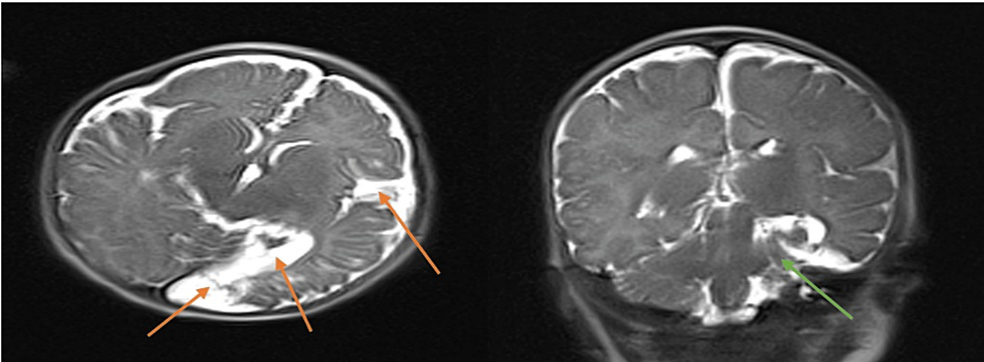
T2, diffusion-weighted magnetic resonance imaging, four weeks after discharge, showing evolution of the left cerebral infarction predominantly involving the left temporal and left occipital lobe with gliosis in this region (green arrow) and increased prominence of the surrounding extra-axial fluid spaces and adjacent left lateral ventricle most likely representing ex vacuo phenomenon. There is no mass effect, midline shift, herniation, or edema. Cerebral volume loss in the left temporal occipital lobes (orange arrows).
